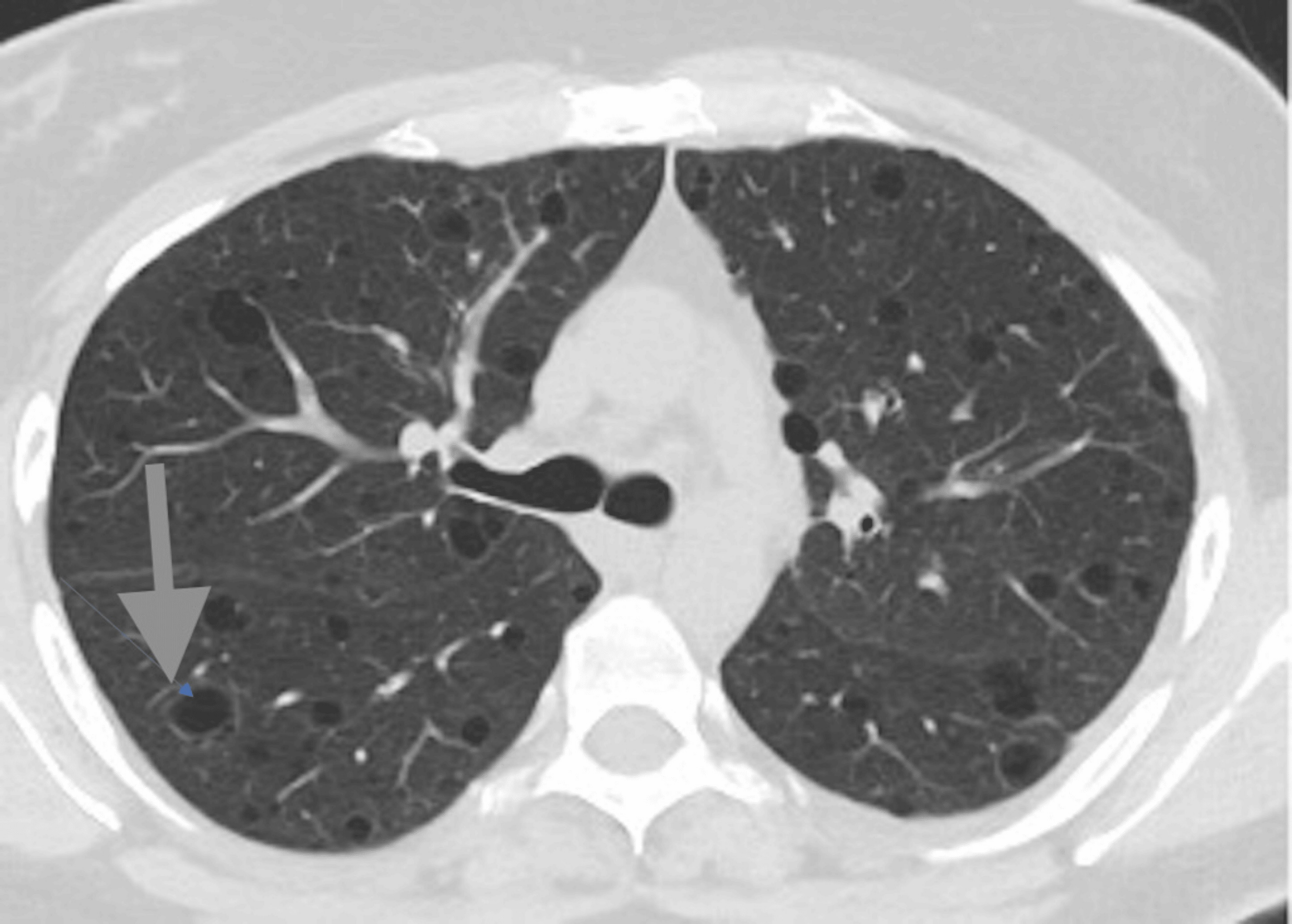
High-resolution computed tomography of the chest HRCT demonstrates innumerable thin-walled cysts distributed bilaterally (arrowheads), characteristic of lymphangioleiomyomatosis. No consolidation or nodules are observed. Image obtained using standard HRCT protocol (1 mm collimation) HRCT - high-resolution computed tomography
